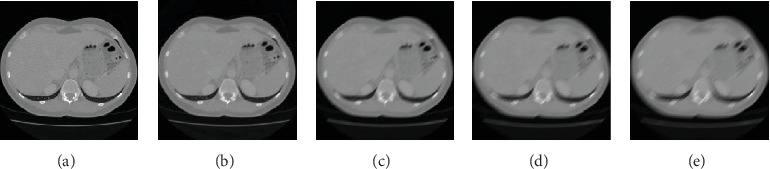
The results of different blurred amplitudes of fatty liver patient. (a) Original CT image. (b) Blurred amplitude of 5. (c) Blurred amplitude of 15. (d) Blurred amplitude of 20. (e) Blurred amplitude of 25.
